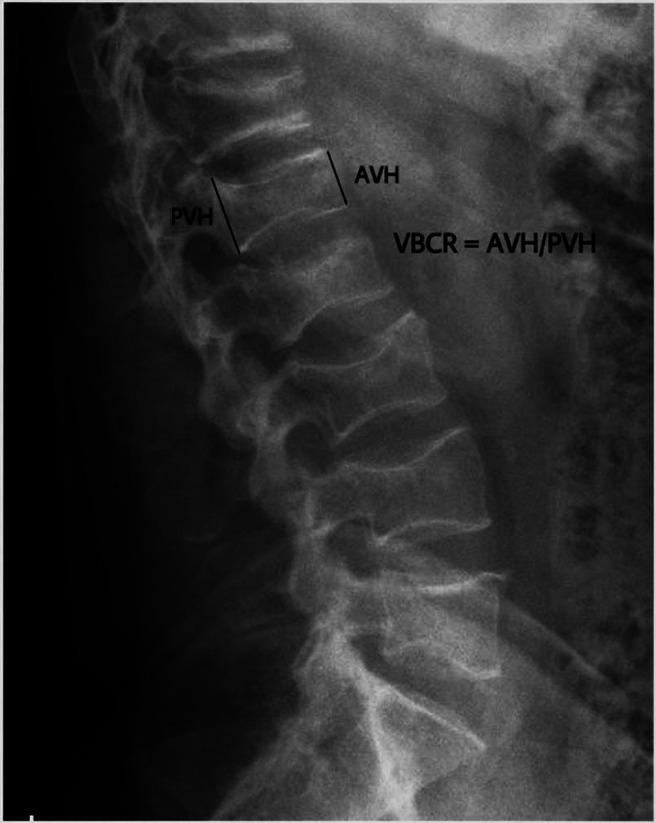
Plain radiographs were obtained before and after the surgery. We used the vertebral body compression ratio (“VBCR”), i.e. the ratio of anterior vertebral height (AVH) to posterior vertebral height (PVH) with the formula VBCR = AVH/PVH. This resulted in a percentage which was used as a parameter for vertebral body height loss.
